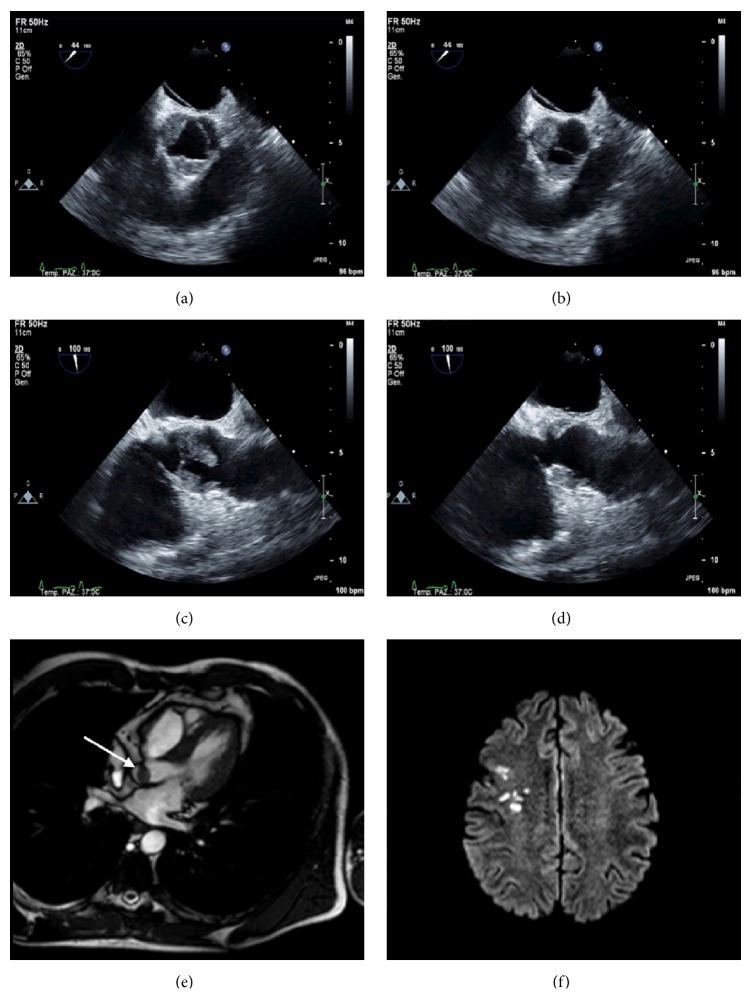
Thrombotic obliteration of the right and no-coronary Valsalva sinuses in systole (a) and diastole (b) in the upper transesophageal short axis view. Floating thrombi attached to the aortic side of the right and no-coronary Valsalva sinuses, extended to the corresponding cusps in diastole (c) and systole (d), in the upper transesophageal long axis view. (e) Cardiac MRI image showing thrombotic mass attached to the aortic valve (arrow). (f) Multiple cortical-subcortical ischemic lesions in the right frontal lobe, at the cerebral MRI.
